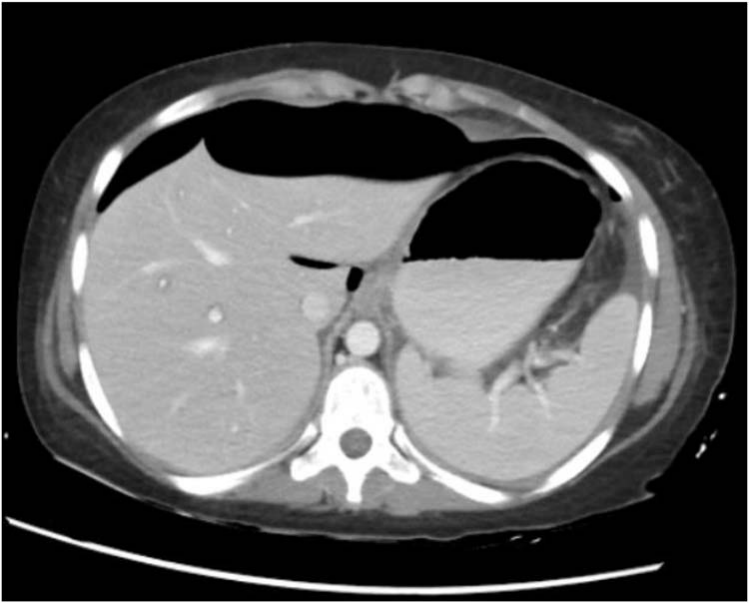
CT abdomen with oral and IV contrast.
